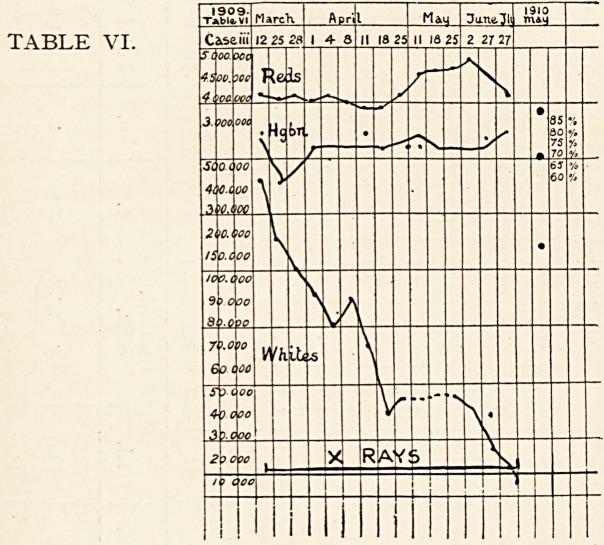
# Cases of Leukæmia Treated by X-Rays
